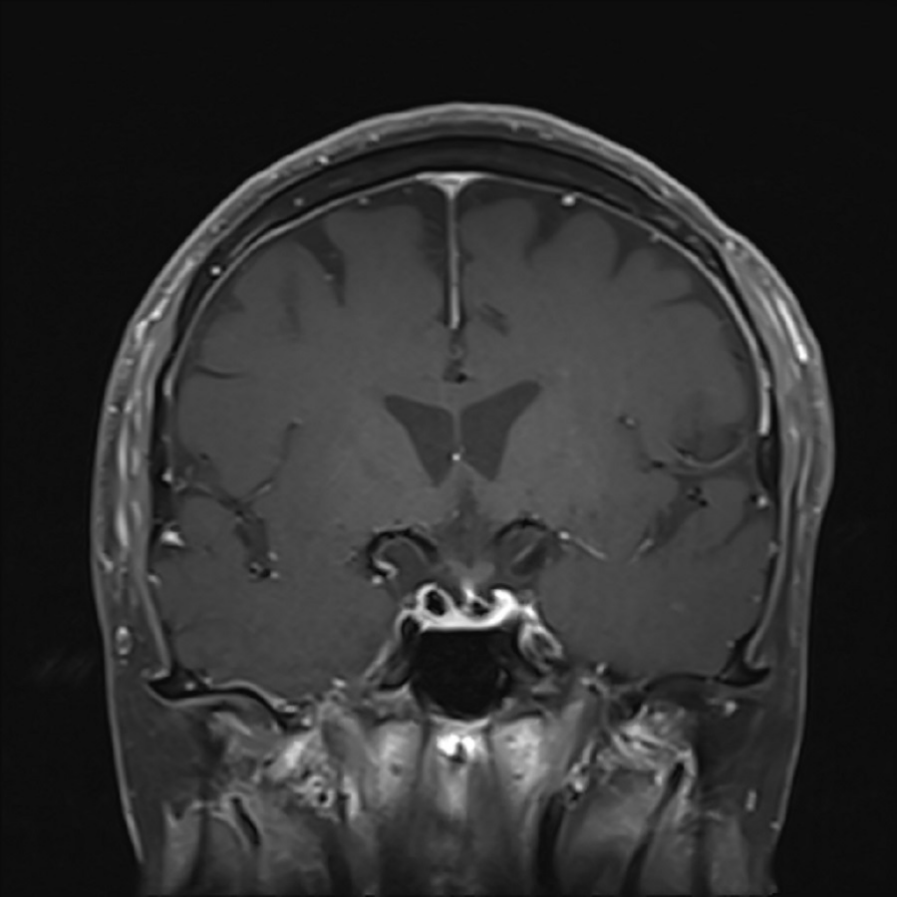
Follow-up MRI obtained approximately three and a half months following initial imaging T1 coronal MRI demonstrating complete resolution of the mass. The infundibulum is now visible and the optic chiasm is in its anatomic position.
